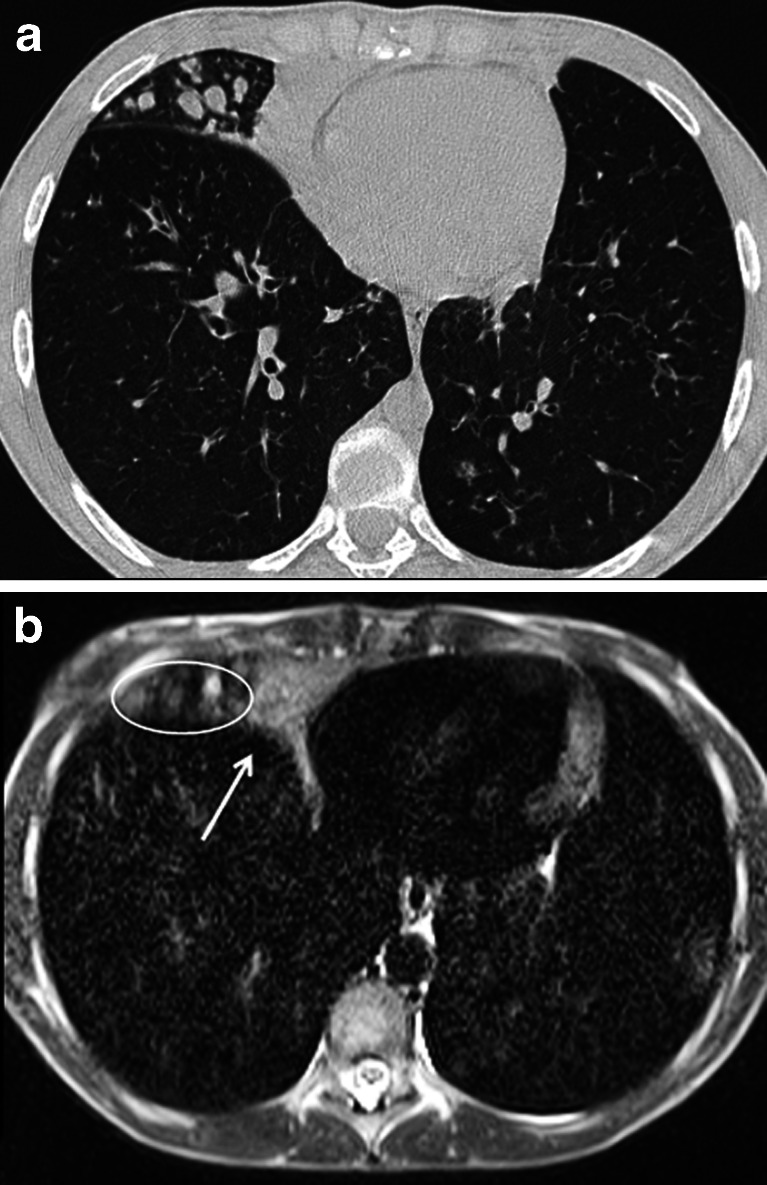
Steady-state free precession MRI in a 12-year-old girl with cystic fibrosis. Comparison between (a) CT and (b) MRI at end-inspiration. Note the consolidation in the right middle lobe (*arrow*) and the adjacent mucus-filled bronchi (*circle*)
